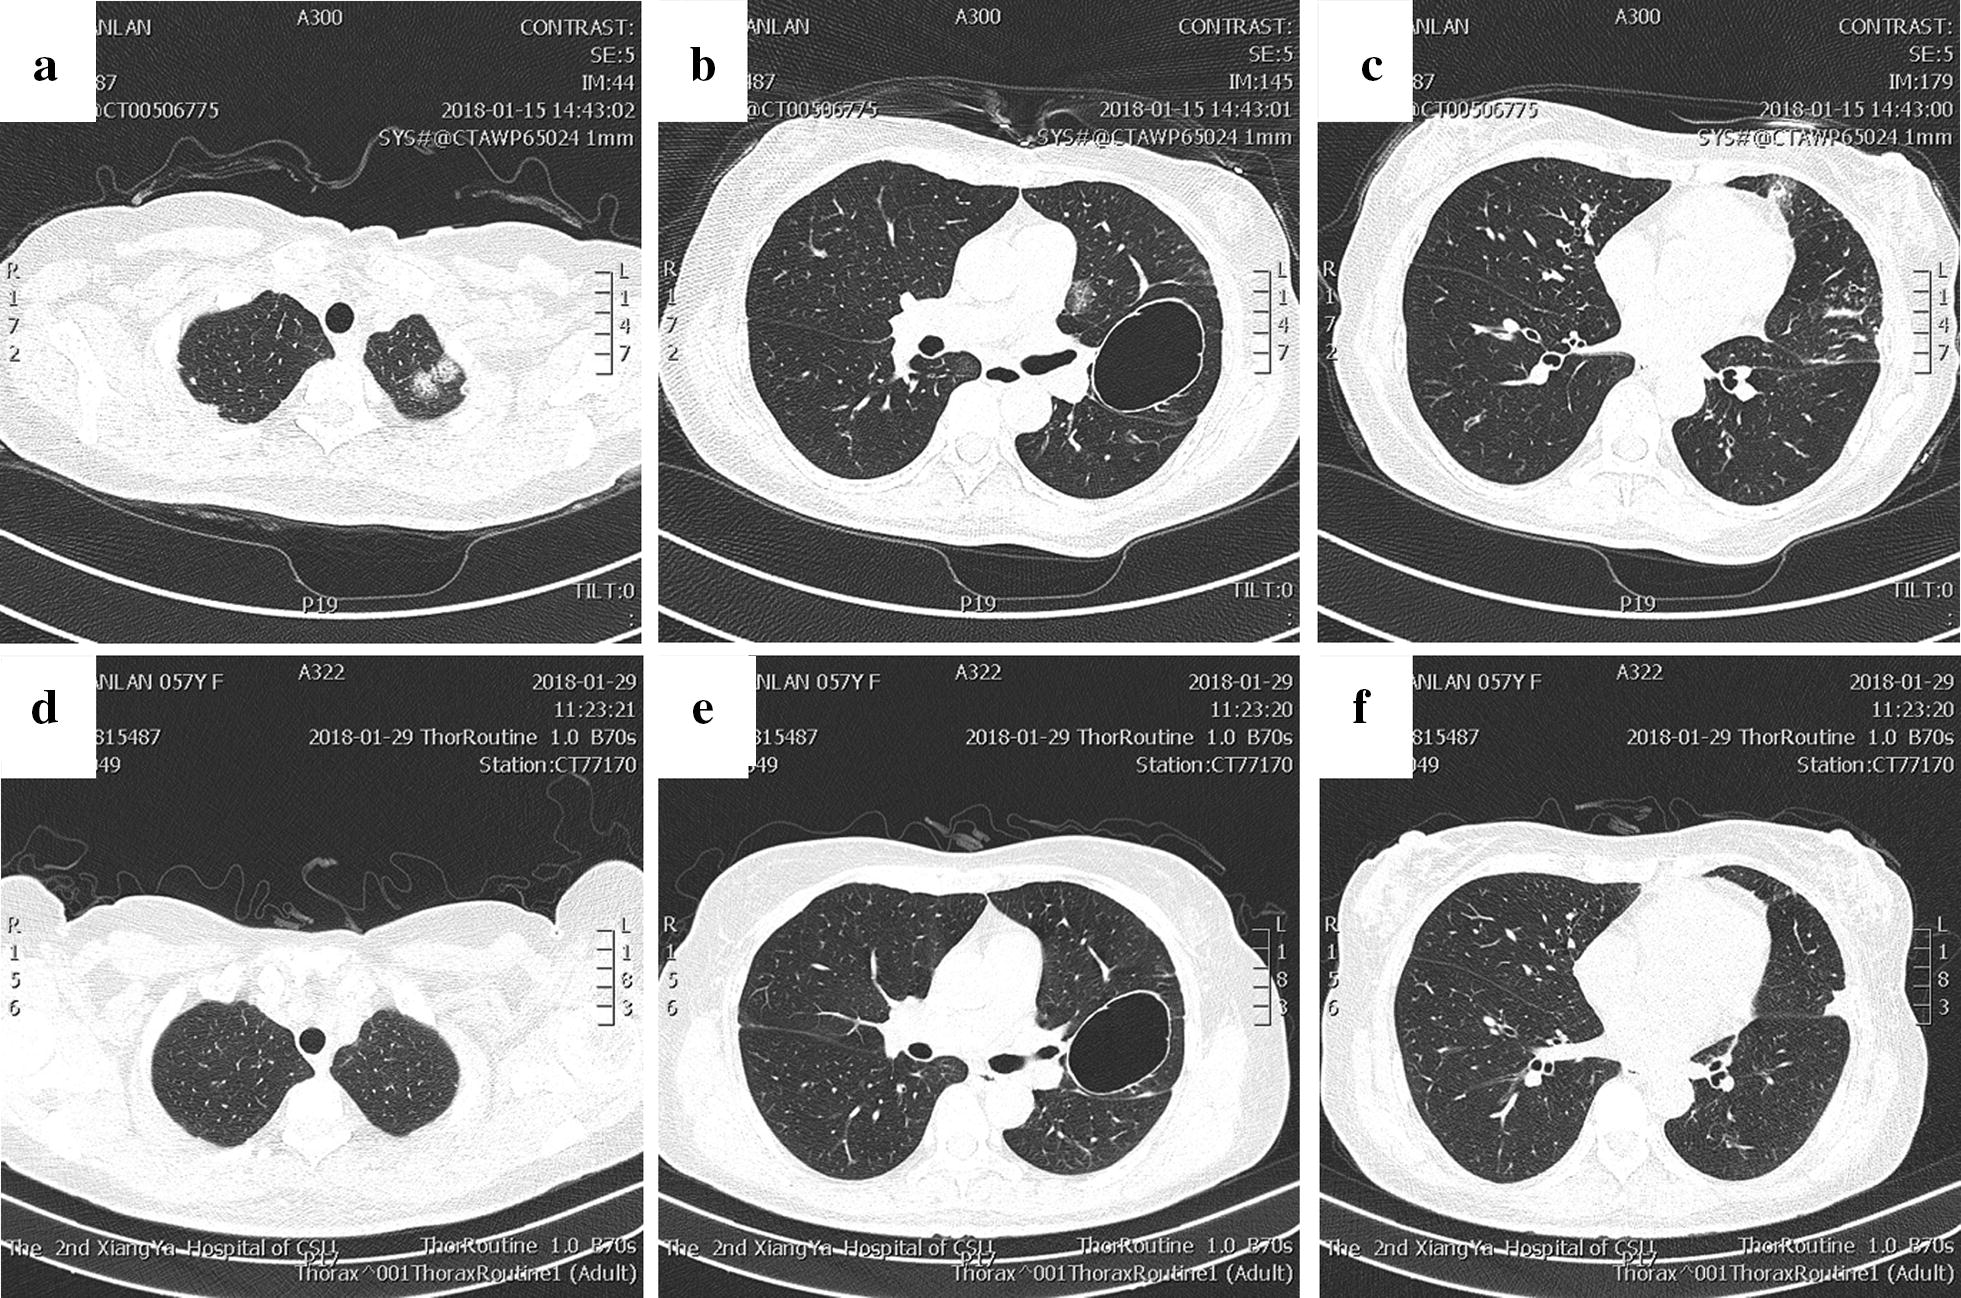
Chest CT images. a–c Chest CT showed multiple infiltrates in the upper left lung and lower right lung and ground-glass opacities in the subpleural regions of both upper lung fields; d–f the lesion in the lower right lobe was partially absorbed
